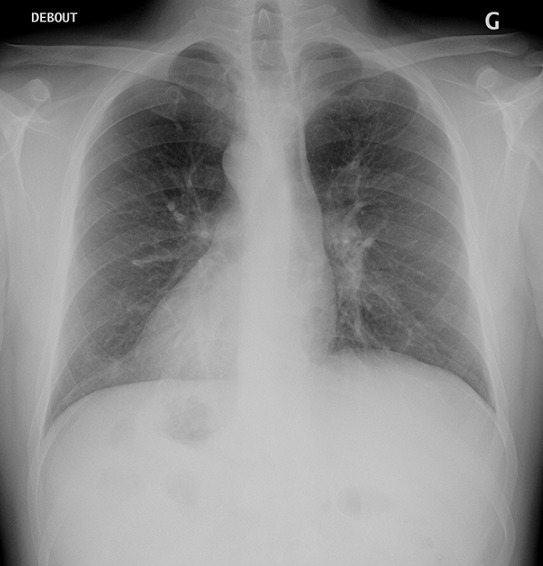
Radiographie thoracique de face: dextrocardie, bouton aortique droit, inversion avec une position en miroir de l'opacité hépatique à gauche et de la poche à air gastrique à droite
